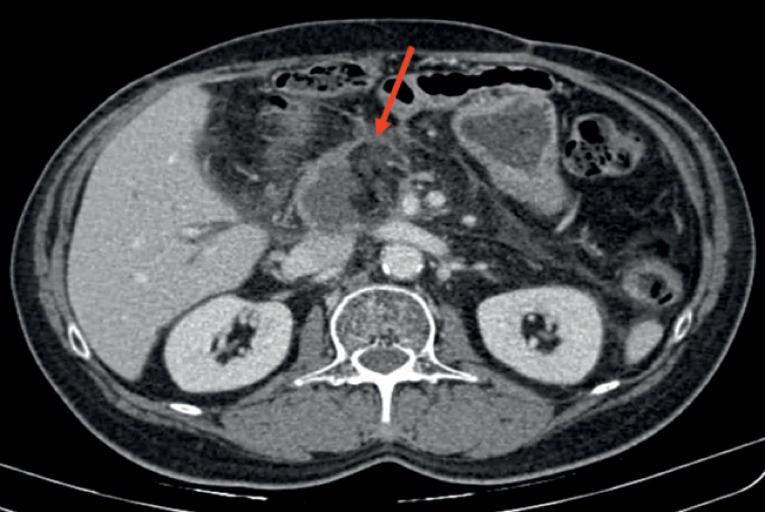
Axial section of contrast-enhanced abdominal computed tomography scan revealed partial absence of the wall of the jejunum loop used for anastomosis with the biliary system (red arrow), suggesting necrosis of this loop.
